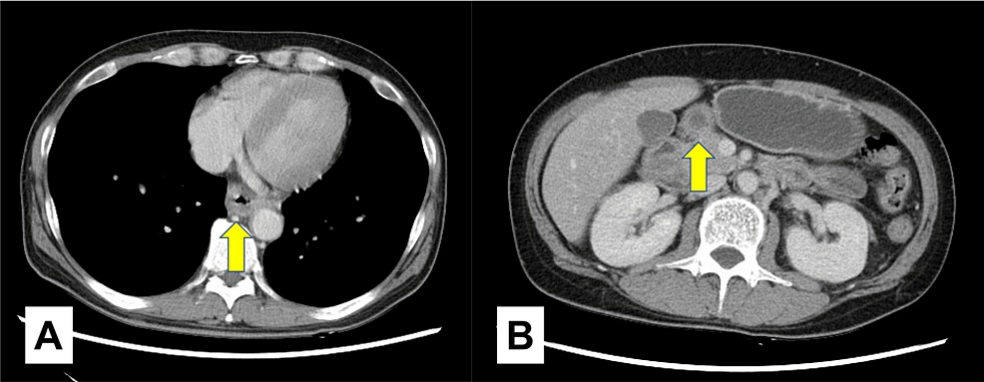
Contrast-enhanced computed tomography of the thorax and abdomen (horizontal section) A: Edematous thickening of the esophageal wall; B: Esophageal and gastric dilatation with pyloric extension
